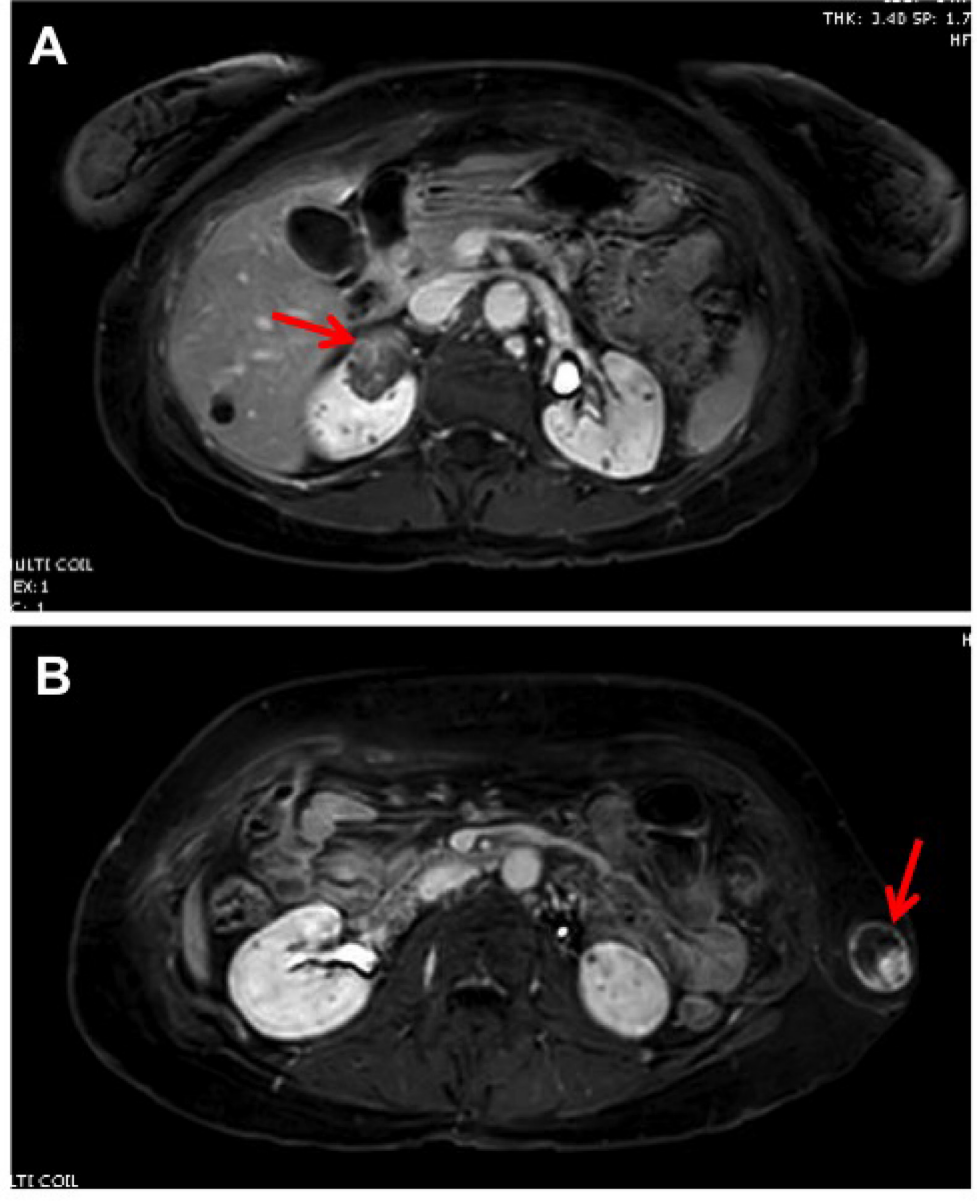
Magnetic resonance imaging (MRI) of the abdomen and pelvis (T1 with gadolinium) demonstrating (A), solid renal mass with radiographic evidence of fat in the upper pole of the right kidney and (B), left flank mass.
